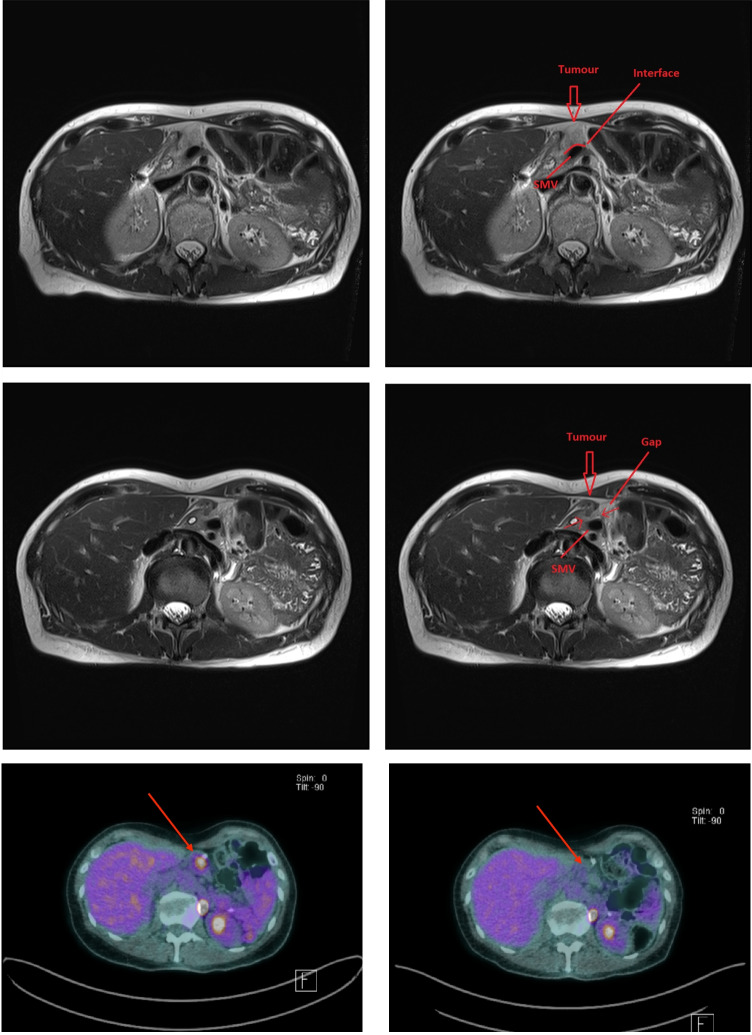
a Axial MRI images in July 2023 prior to treatment (top panels) and in October 2023 after 4 cycles of Pembrolizumab (bottom panels). Images demonstrate initial tumour contact with the SMV and a tissue plane after treatment. b Axial PET-CT image in July 2023 prior to treatment (left) and in October 2023 after 4 cycles of Pembrolizumab (right). A high-FDG avid lesion is seen in the pancreatic head region (arrow) with a complete metabolic response after treatment
